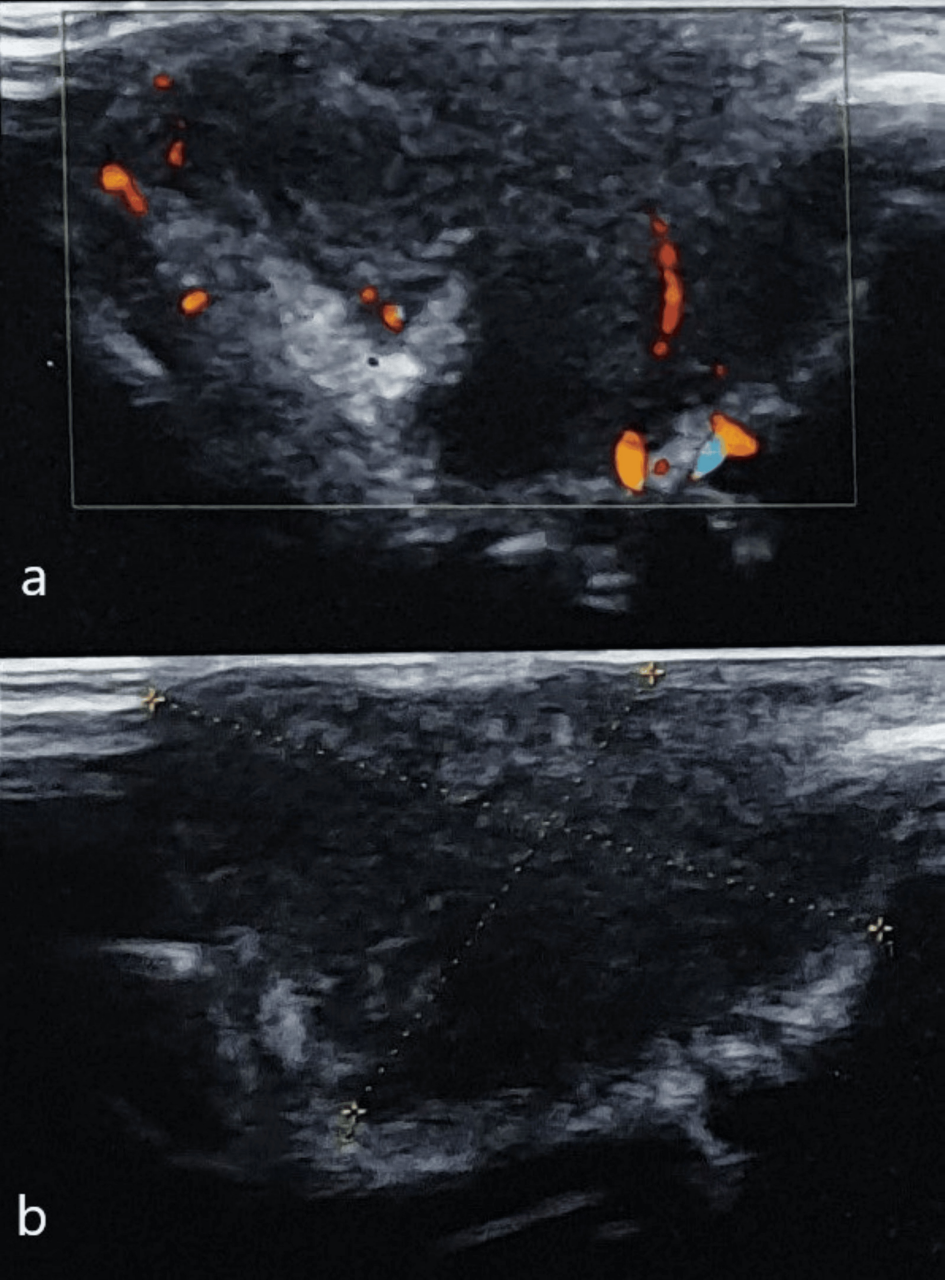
Ultrasound B scan showing (a) hypoechoic soft tissue mass lesion along the left lower orbital margin in the medial aspect and (b) regression of lesion after six weeks
